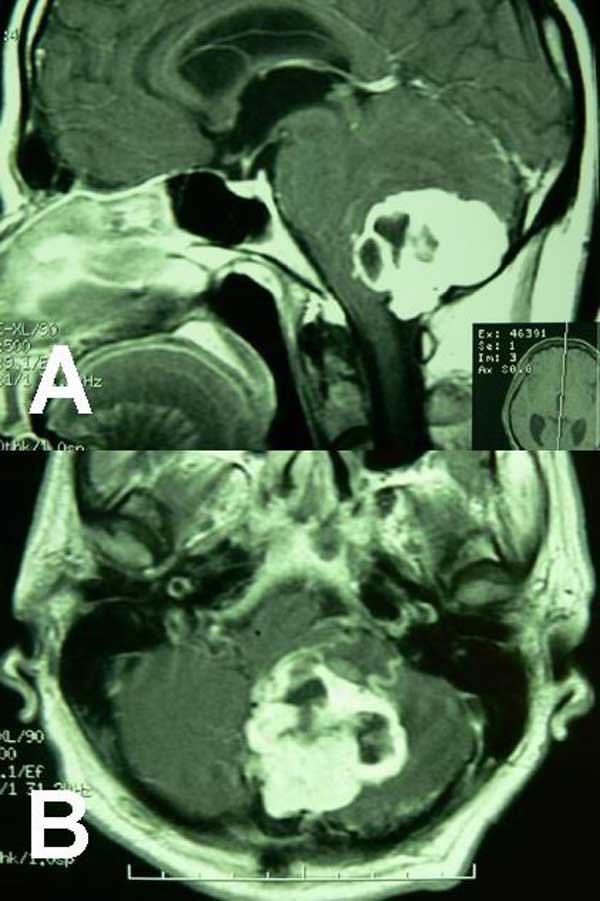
(A) and (B) Sagittal and axial T1-weighted magnetic resonance images of brain after contrast injection showing a solid-cystic densely enhancing mass in the left cerebellar hemisphere with compressive effect upon the fourth ventricle causing obstructive hydrocephalus.
